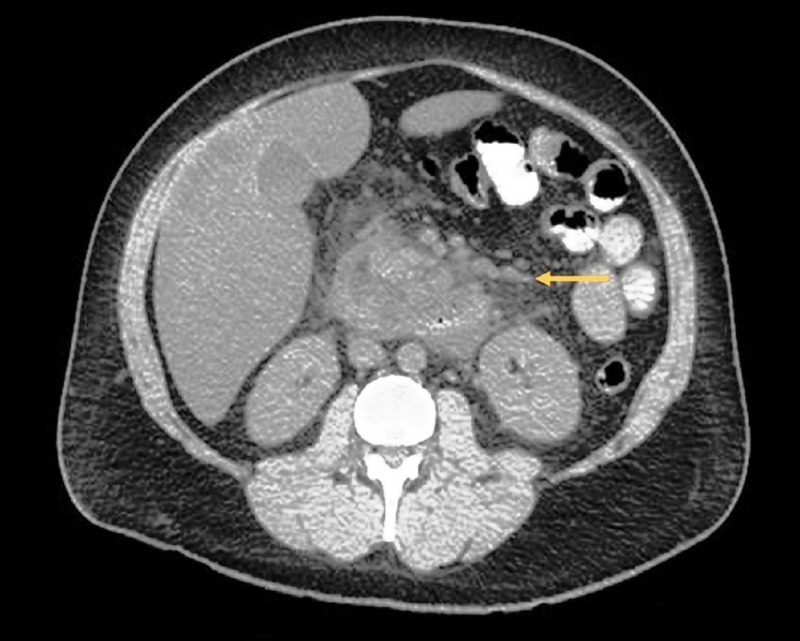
Computed tomography abdomen showing enlarged and edematous pancreas with smooth, indistinct margins, nonvisualized pancreatic duct, and peripancreatic fat stranding. Arrow indicates the above-mentioned findings.
